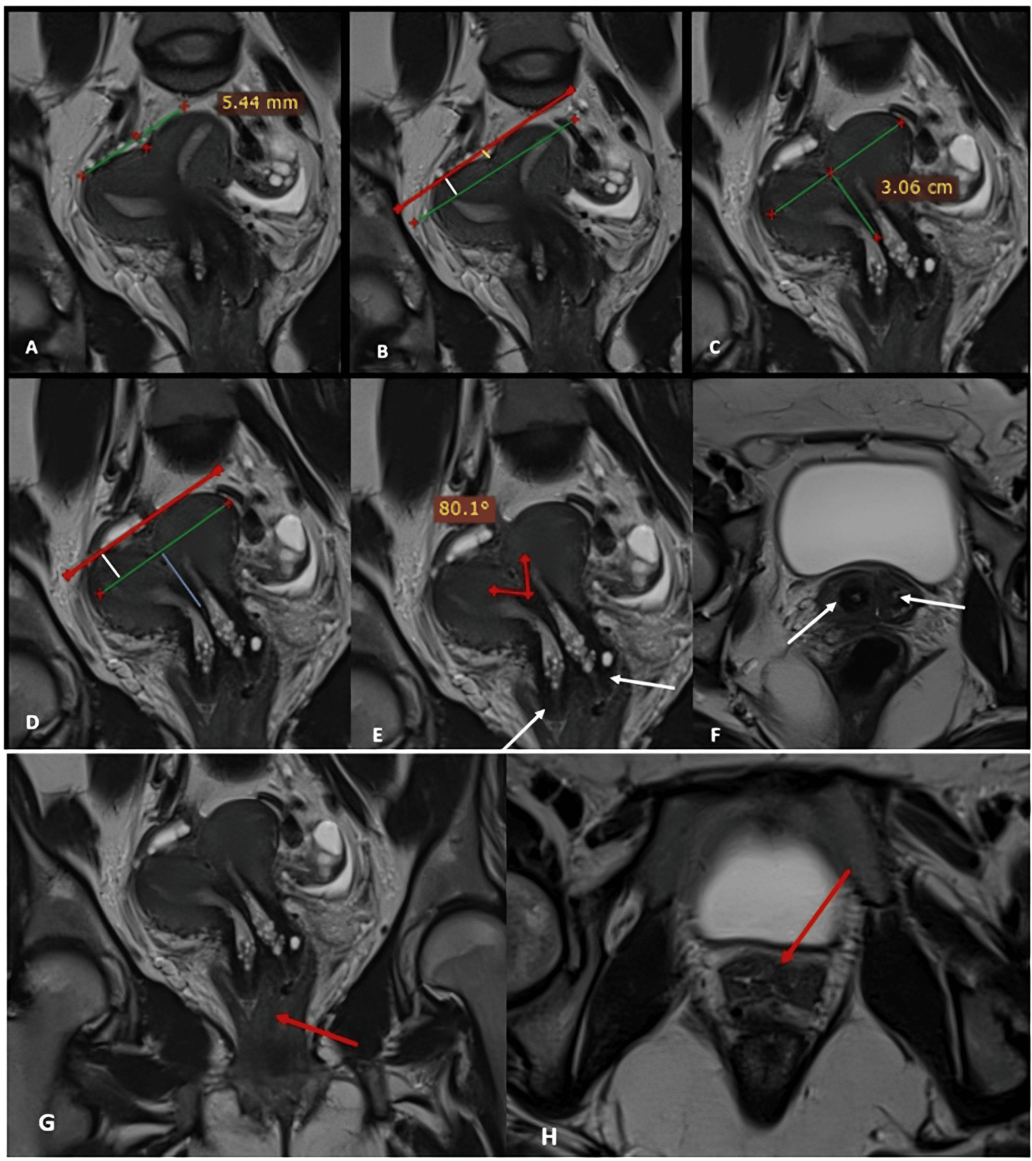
Pelvic MRI in T2-weighted coronal and axial sections showing a septate uterus (A-E), duplicated cervix (E, F; white arrow), and longitudinal vaginal septum (G, H; red arrow) MRI: magnetic resonance imaging
